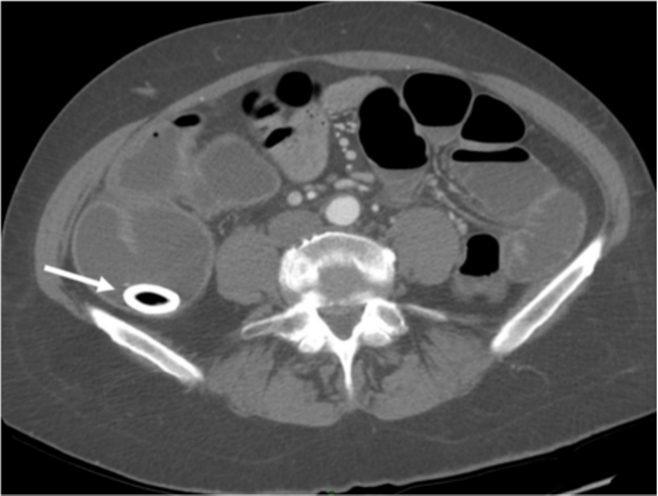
Computed tomography scan showing the stone in the terminal ileum. Arrow indicates gallstone.
